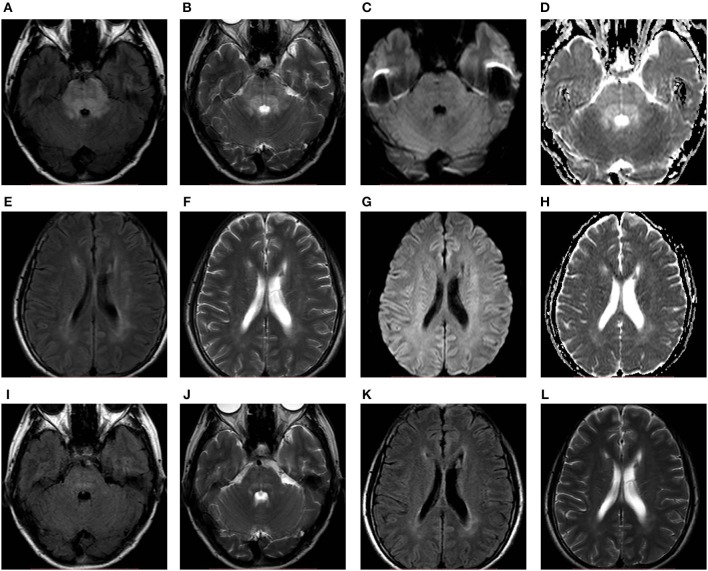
A 52-years-old female with hypertension presented with headache. FLAIR (A,E), T2WI (B,F), and ADC maps (D,H) showed hyperintensity predominantly in the brainstem accompanied by periventricular white matter. No obvious abnormality on DWI (C,G). After 11 days of follow-up, the abnormal signals (I–L) markedly resolved. ADC, apparent diffusion coefficient; DWI, diffusion-weighted imaging; FLAIR, fluid-attenuated inversion recovery; T2WI, T2-weighted imaging.
